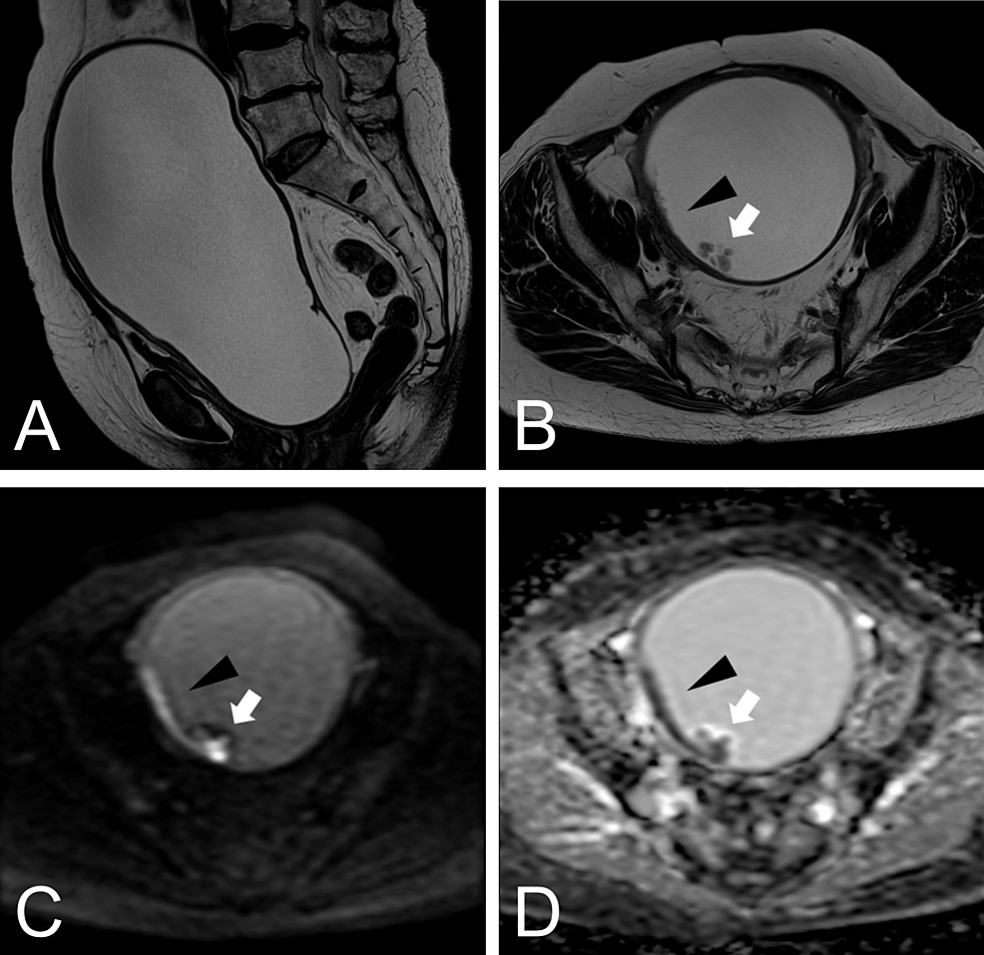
MRI findings (A) Sagittal, T2-weighted MRI section showed an unilocular cystic lesion. (B) Axial T2-weighted MRI. (C) DWI and (D) ADC maps showed a thickened wall (black arrowhead) and mural nodule (white arrow). MRI, magnetic resonance imaging; DWI, diffusion-weighted imaging; ADC, apparent diffusion coefficient
